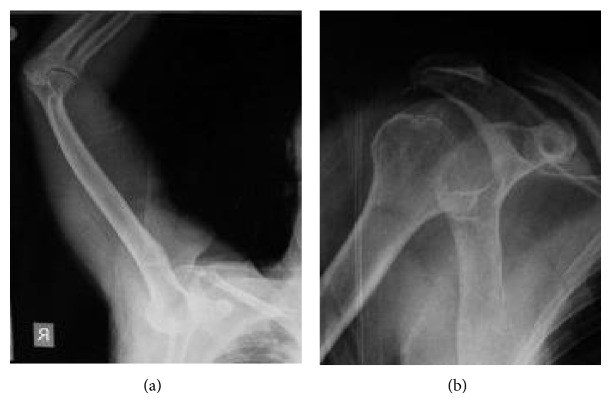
X-ray of the humeral head located below the rim of glenoid and X-ray after the reduction was achieved.
